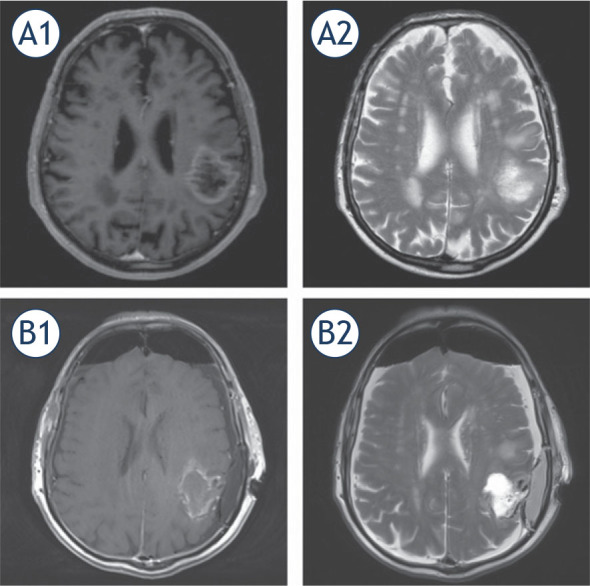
Preoperative (A) and postoperative (B) T1 weighted contrast enhanced (A1, B1) and T2 weighted (A2, B2) magnetic resonance images (MRI) of glioblastoma located in supramarginal gyrus near Wernicke area. Preoperative images (A) demonstrate rim enhancing central necrotic oval lesion surrounded by oedema. Postoperative images (B) demonstrate post-resection cavity filled with partially haemorrhagic fluid and mild irregular contrast enhancing of resection edge – i.e. near total resection.
